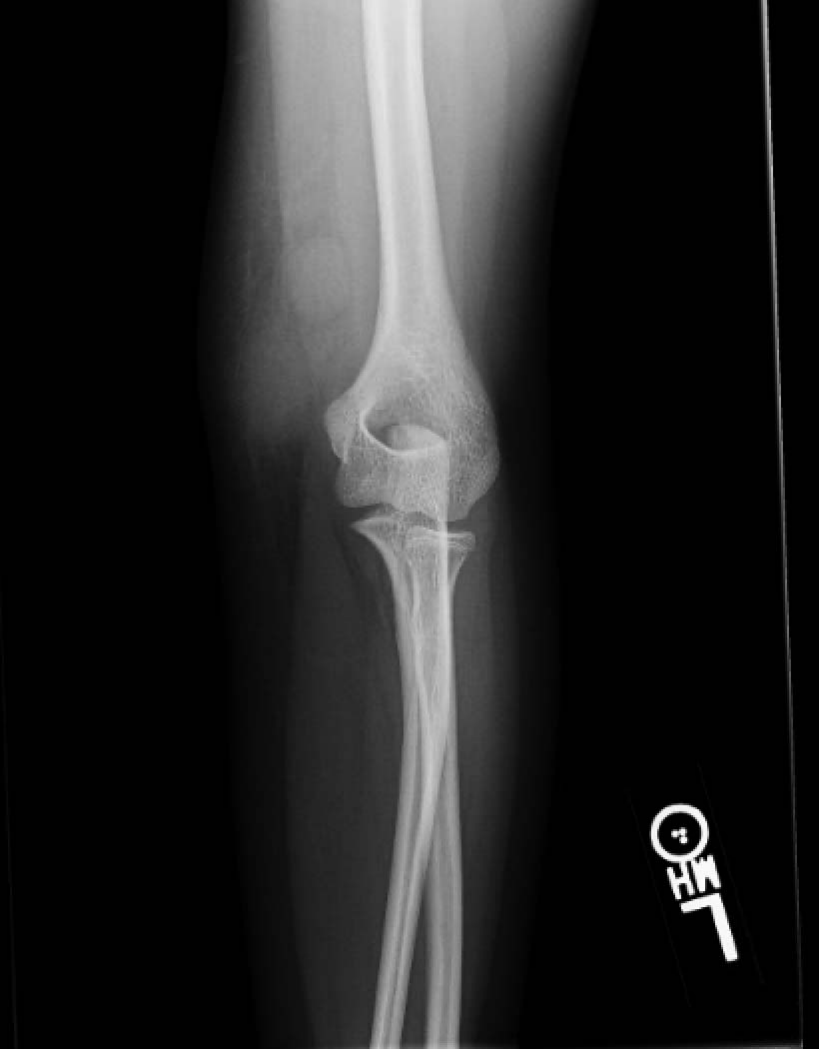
Radiograph of the left elbow shows no acute fracture process or significant edema.
